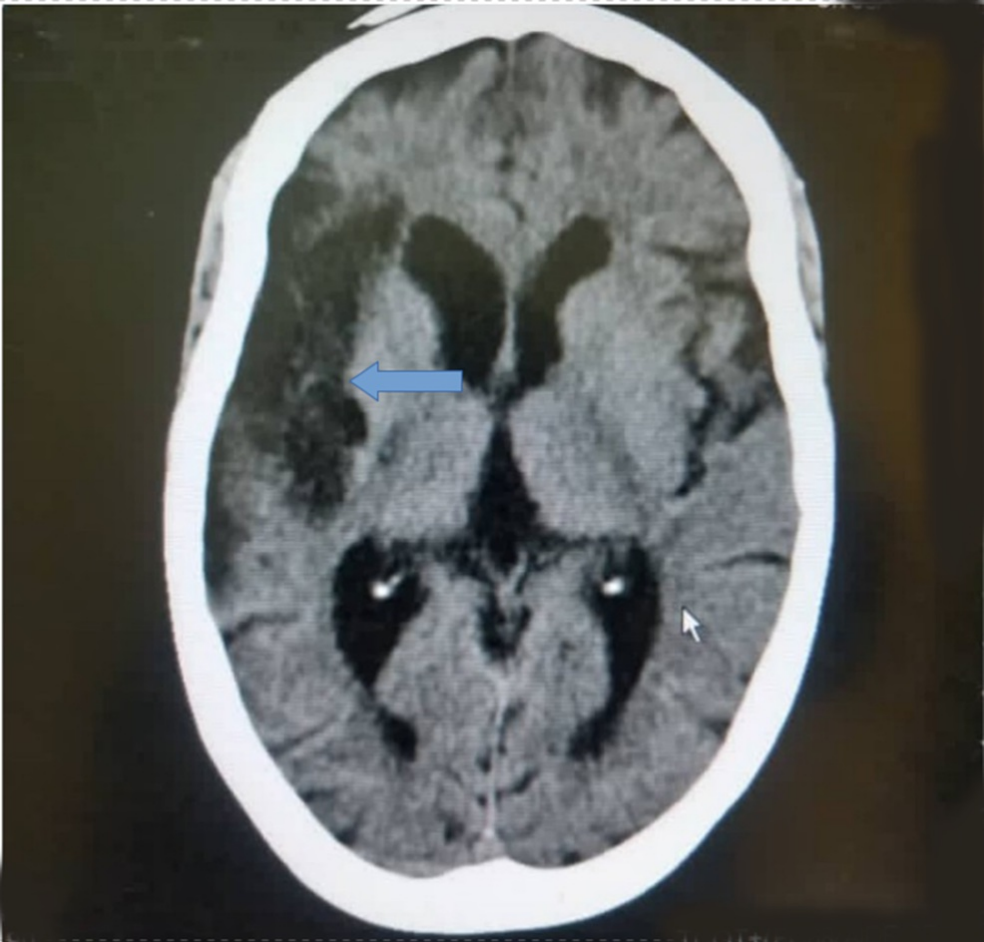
An axial non-enhanced CT scan of the brain of a 56-year-old man, hypertensive for 12 years, seen with a sudden onset of left hemiplegia and aphasia, showing an extensive area of hypodensity in the region of the right temporoparietal brain (blue arrow) with a comparative dilatation of the anterior horn of the ipsilateral lateral ventricle in keeping with right temporoparietal chronic infarct.
